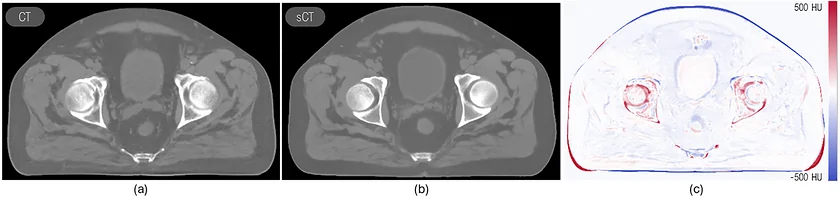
Image comparison. Figure showing in a slide from the CT in (a) and sCT in (b). The difference between CT and sCT is seen in (c).
